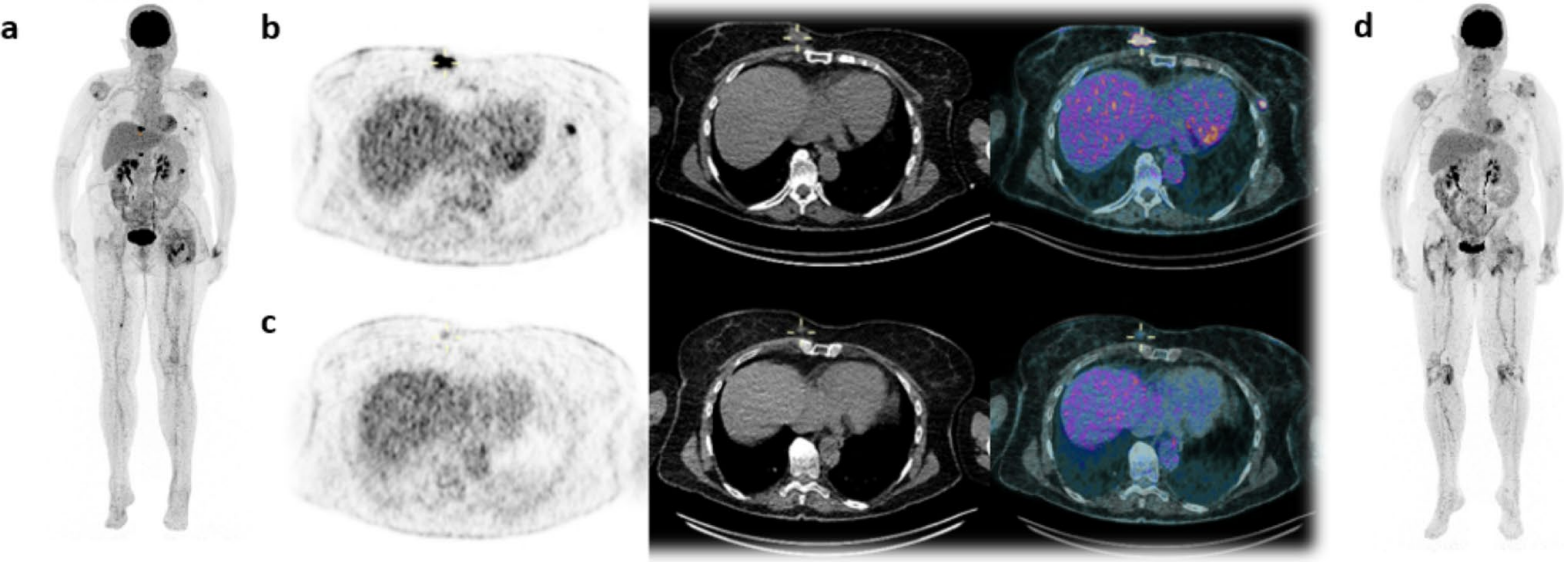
80-year woman with stage IV melanoma with unknown primary with multiple metastatic lesion: a and d) Maximum intensity projections [^18^F]FDG PET images for the first scan and second scan at three months, respectively; b) transaxial slices showing PET, low-dose CT and fused PET/CT images of the first [^18^F]FDG PET/CT scan, and c) transaxial slices showing PET, low-dose CT and fused PET/CT images of the second [^18^F]FDG PET/CT scan with a partial metabolic response
